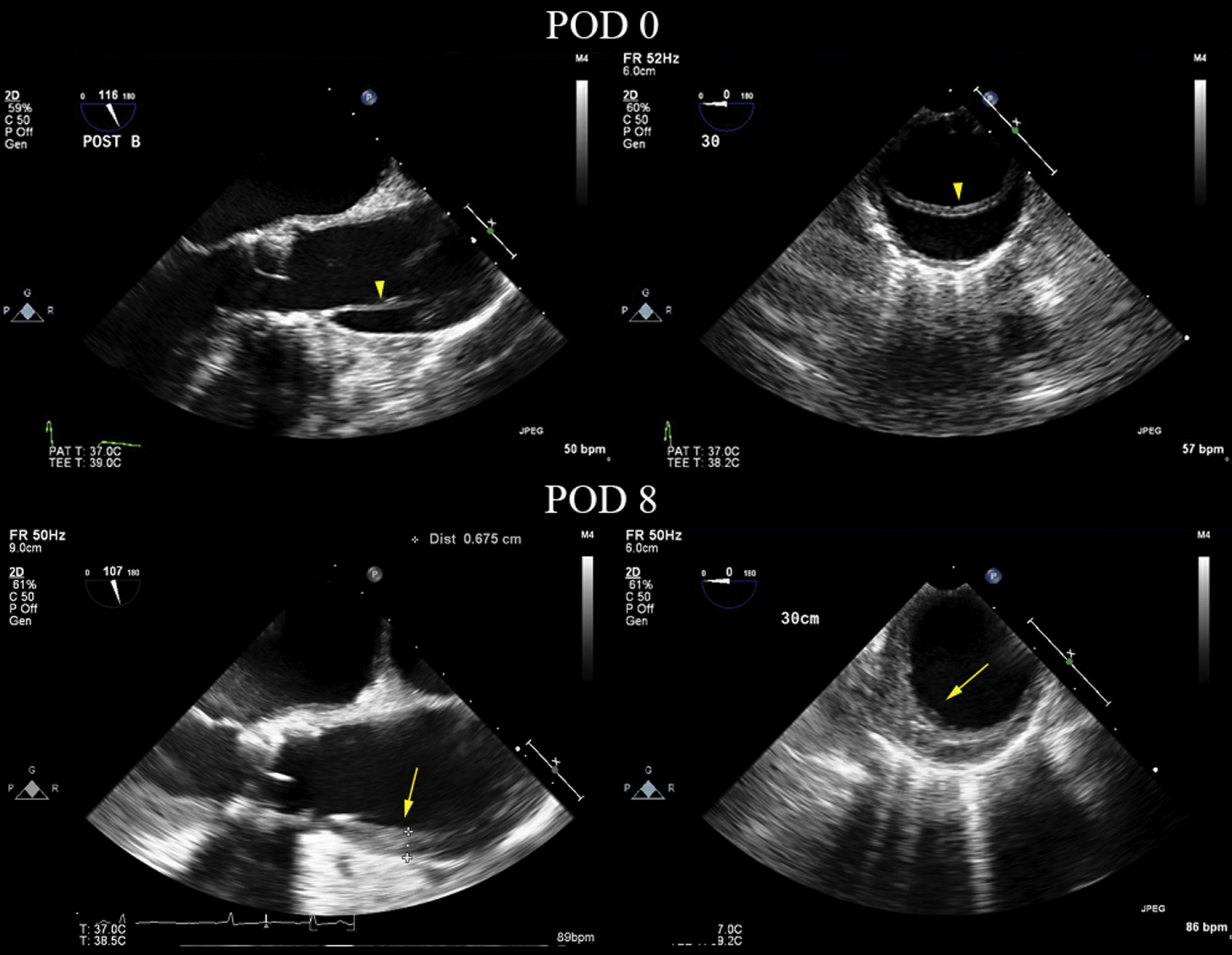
Serial Follow-Up of the Transesophageal Echocardiography Images Serial follow-up transesophageal echocardiography images show the intimal flap (arrowheads) originating from the aortic root after device implantation and a compactly thrombosed false lumen (arrows) on postoperative day (POD) 8.
